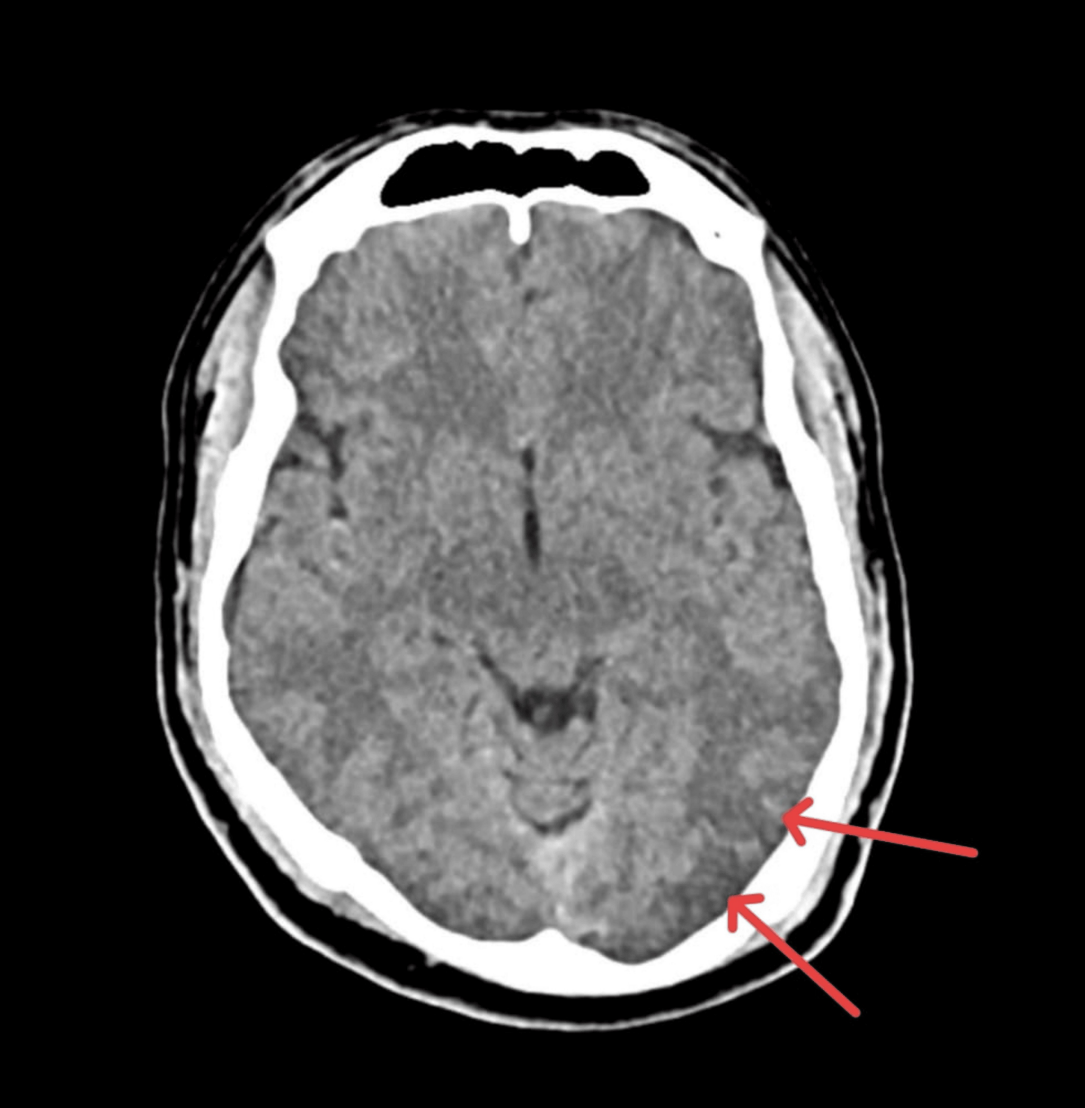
Axial CT of the brain showing subtle cerebral oedema in the left parieto-temporal lobe (red arrows). CT: computed tomography
